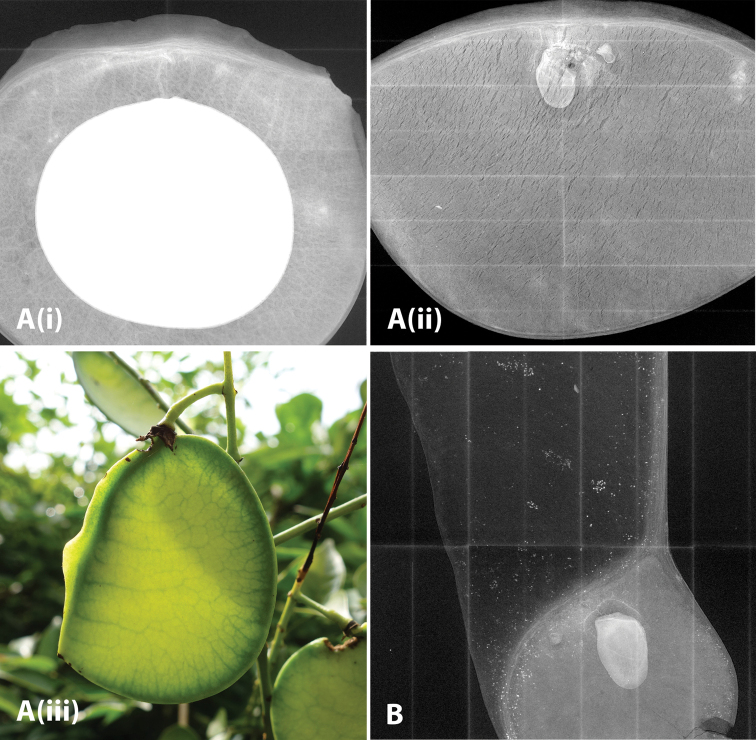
Fruit wing venation A*T.sinensis* fruit with wing (i) (X-ray) (*Z. Zhang 148*, China (MO)) (ii) (X-ray) (*W.E. Qun 150*, China (K)) (iii) (photo by R.P. Clark, Guangxi, China, *unvouchered*) B*Pterolobiummicrophyllum* fruit with wing (X-ray) (*C. Phengkhlai 682*, Thailand (K)).
